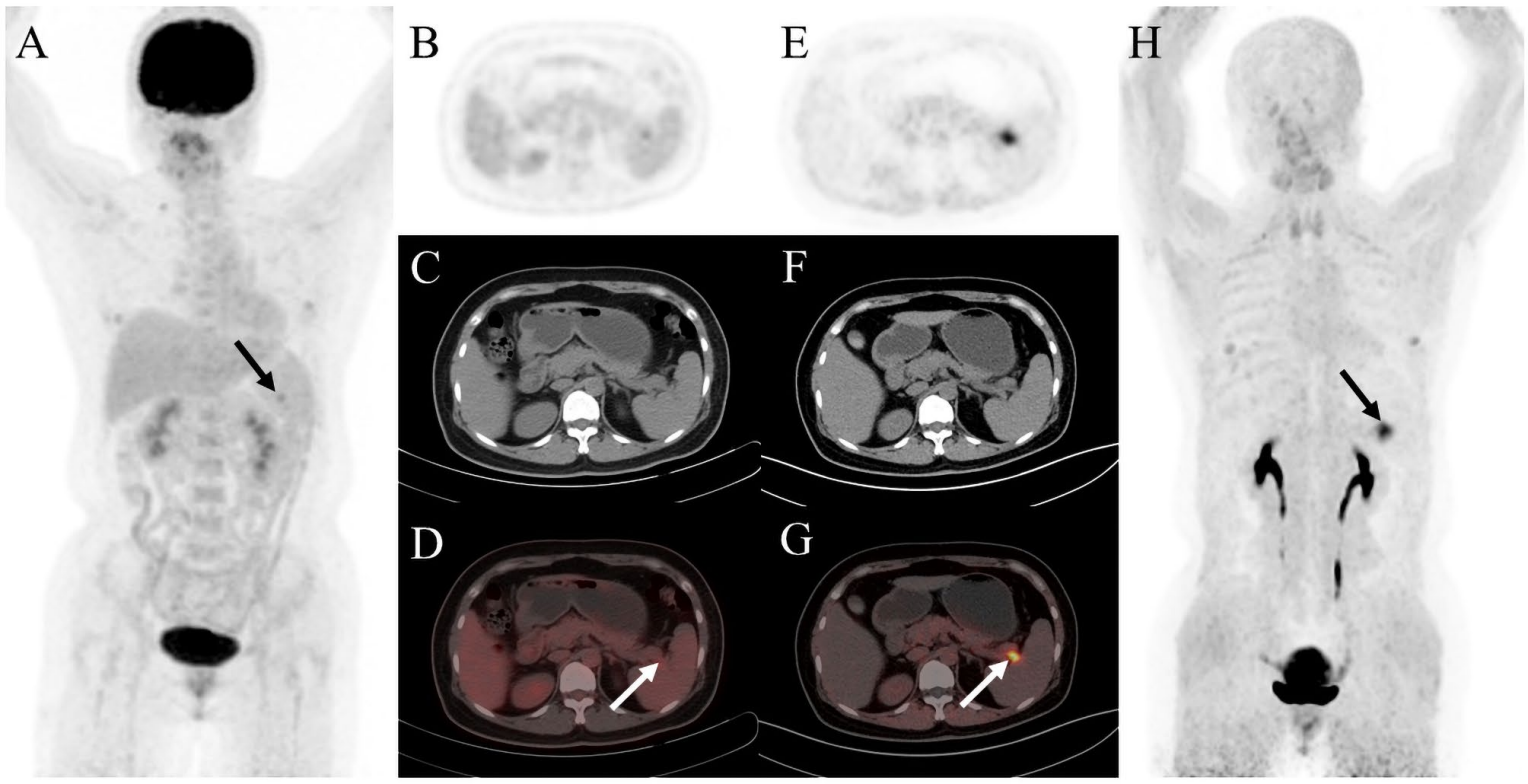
A 45-year-old woman was admitted to our hospital because of bleeding after intercourse for one month. A subsequent cervical biopsy indicated cervical adenocarcinoma. For staging, the patient underwent ^18^F-FDG PET/CT. The MIP image (A) and the axial views (B: PET image; C: CT scan; D: PET/CT fused image) revealed no abnormal uptake. Subsequently, ^68^Ga-FAPI PET/CT was performed. Spleen with intense uptake (E: PET image; F: CT scan; G: PET/CT fused image; H: MIP, solid arrow; SUV_max_, 8.2) was observed on ^68^Ga-FAPI PET/CT. Subsequently, spleen metastasis was confirmed by pathological result
